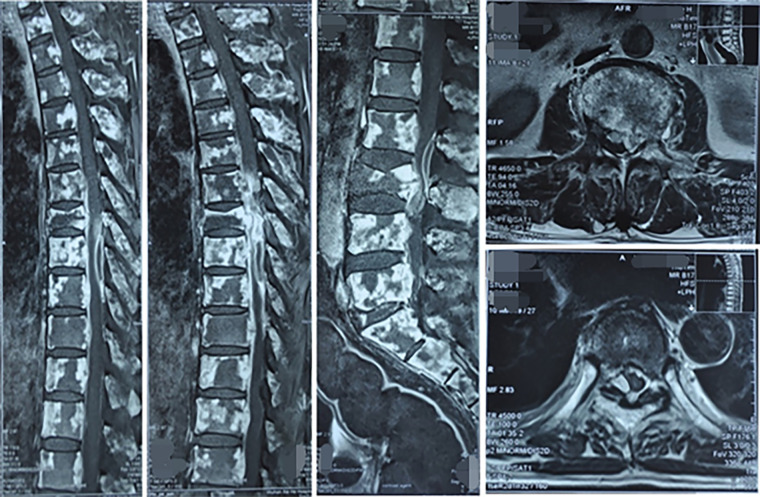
MRI images of vertebral bone metastases and spinal cord compression in primary lung cancer.
